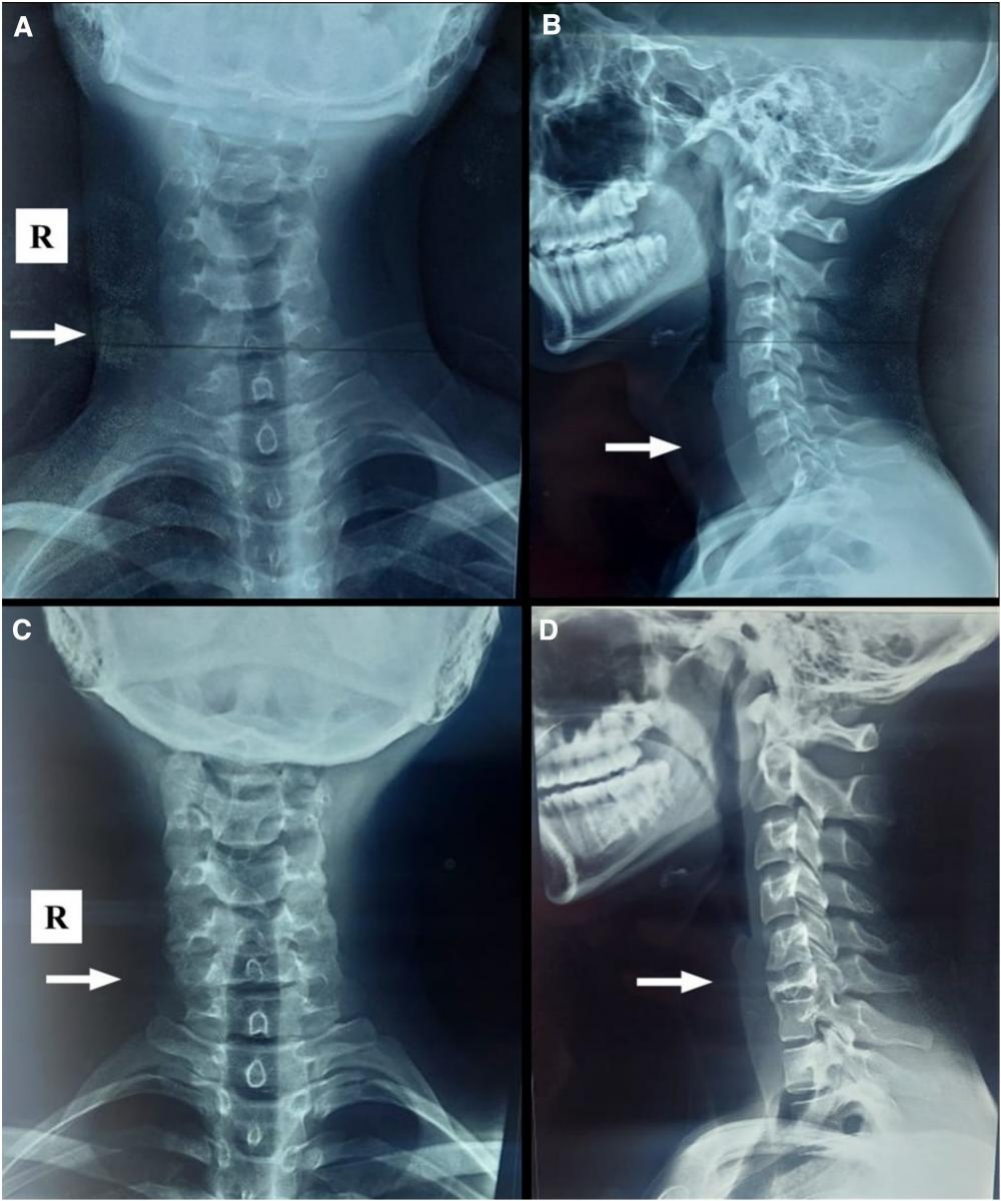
Cervical spine X-ray in anteroposterior and lateral views, demonstrating the progression from normal findings to a C6 vertebral body fracture. (A) Anteroposterior radiograph of the cervical spine obtained at initial presentation, showing normal alignment and vertebral morphology without evidence of fracture or degeneration. (B) Corresponding lateral view demonstrating intact vertebral bodies, preserved disc spaces, and normal cervical curvature. (C) Follow-up anteroposterior radiograph obtained 2 years later, revealing significant compression and degeneration of the C6 vertebral body. (D) Corresponding lateral view showing marked collapse and deformity of C6, consistent with vertebral compression fracture. White arrows indicate the C6 vertebral level in all images. R is for right side.
